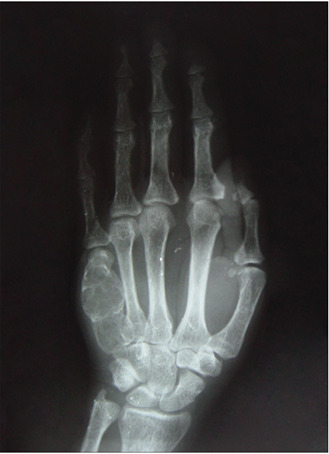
Hand radiograph showing subperiosteal bone resorption at the radial aspect of the middle phalanges, with loss of definition of the peripheral cortex; and a brown tumor, consisting of a cystic, expansible, well-defined lesion, with the cortex thinned and expanded but not penetrated, in the fifth metacarpal.
